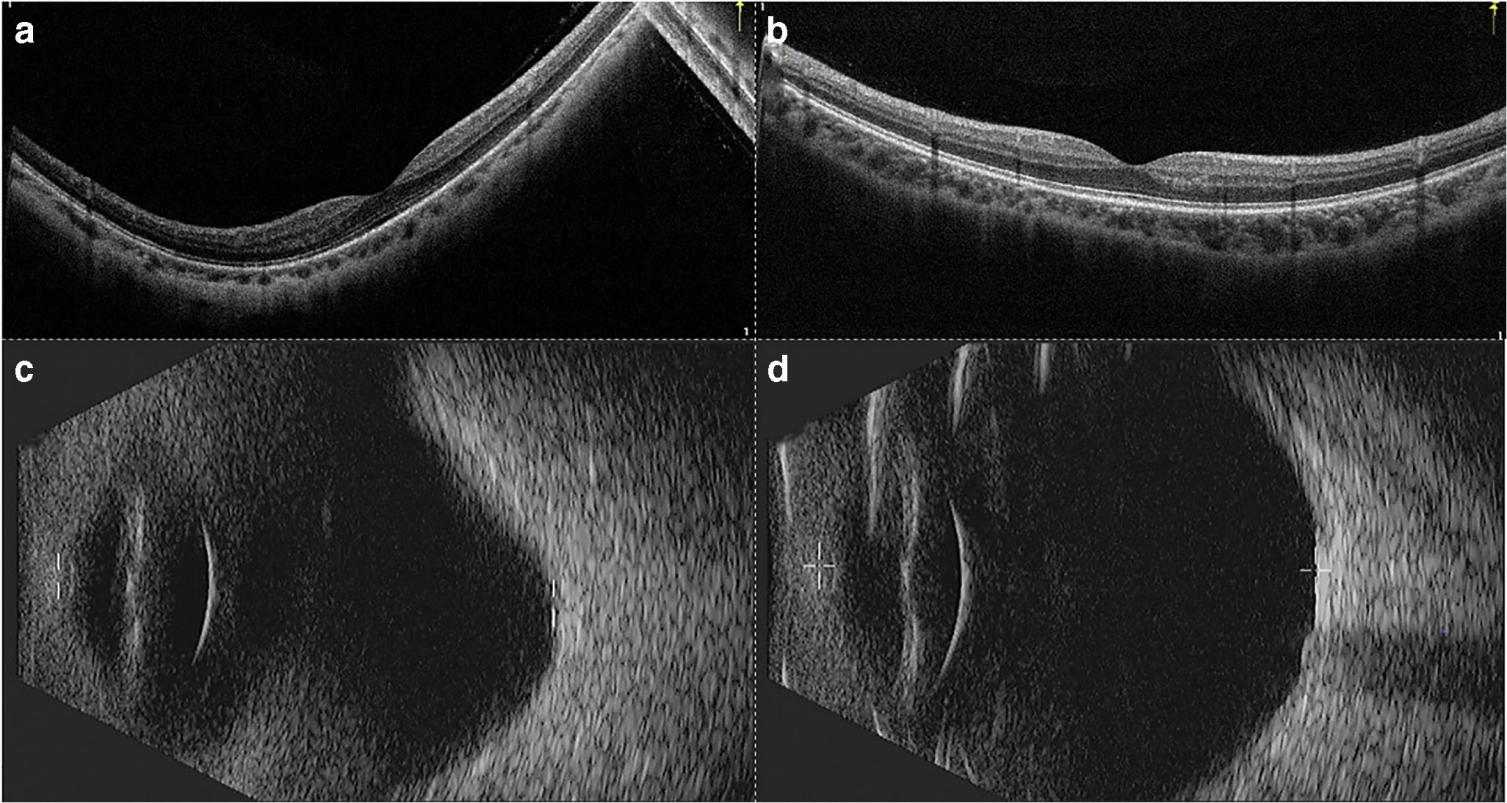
OCT and ultrasonographic images of PS and NPS. a,c An eye with an axial length of 26.76 mm that has PS. b,d An eye with an axial length of 26.76 mm without PS
